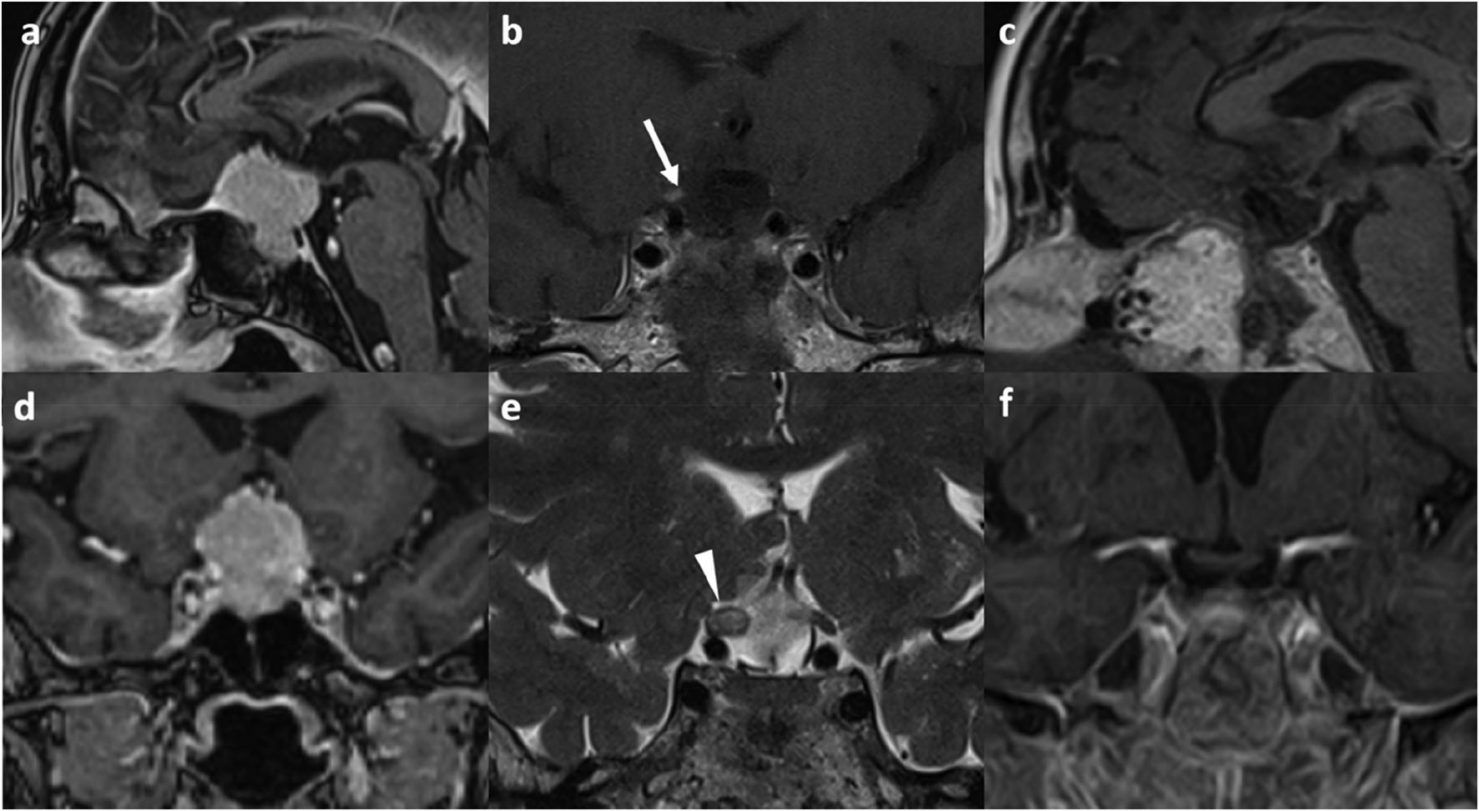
Tuberculum sellae meningioma WHO grade I (*case 1*). a, d Contrast-enhanced MRI. There was invasive tumor growth into the right optic nerve (see Video). b Contrast-enhanced T1-weighted image shows enhancement in the right optic nerve (arrow) and e optic nerve swelling on T2-weighted image (arrowhead). Otherwise, there was no tumor residual (c–f). Pituitary function was preserved. Initially, the patient presented with acute onset visual decline on the right eye and hemianopia. Visual function of the right eye was marginal and could not be preserved. Vision improved immediately on the left eye
